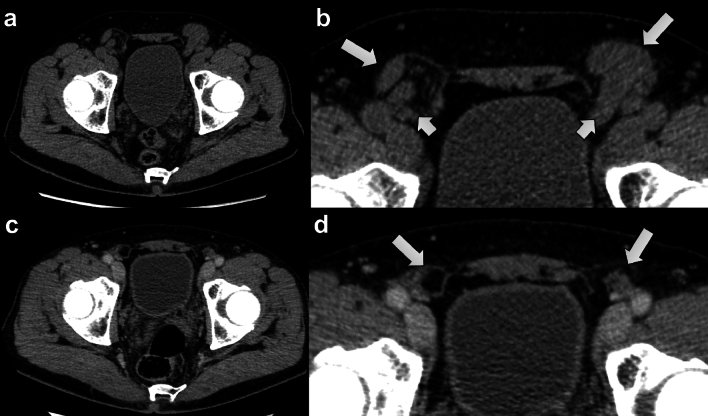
58-year-old male patient with bilateral inguinal hernias. Representative Valsalva CT image (A) and corresponding magnified image of the groin (B) shows bilateral inguinal hernias (long arrows in B) containing bowel loops (short arrows in B). On a non-Valsalva CT scan done 1 month later for pancreatitis (C, and magnified image of the groin in D) the hernias are barely visible (long arrows in D) containing only minimal fat
